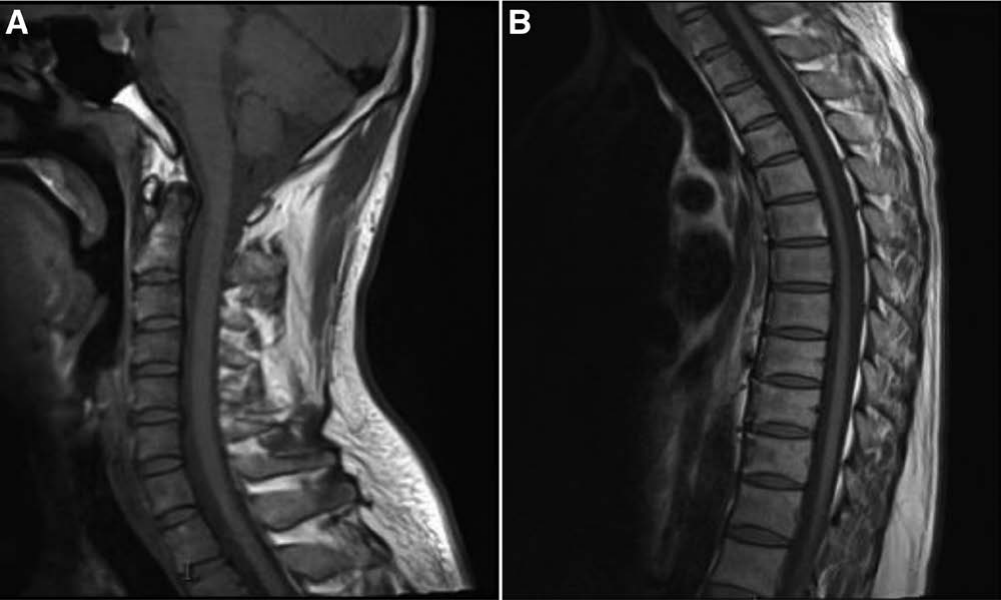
Spinal magnetic resonance imaging (MRI) of the patient without abnormal signal in the cervicothoracic medulla. (A) Mild posterior protrusion of the cervical 5 to 6 disc, (B) mild atrophy of the thoracic medulla.
